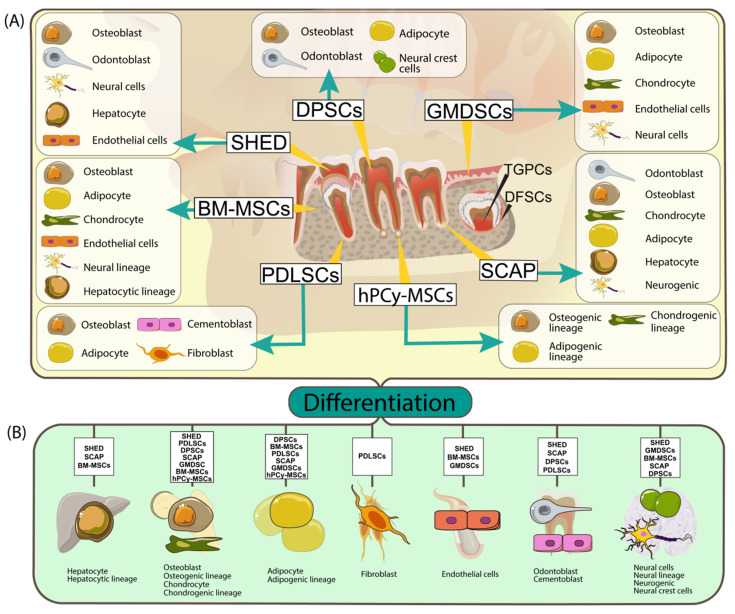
(A) Summary of dental stem cells types, sources and their multilineage differentiation capability (BM-MSCs: bone marrow mesenchymal stem cells; DPSCs: human dental pulp stem cells; SHED: human exfoliated deciduous teeth; PDLSCs: periodontal ligament stem cells; SCAP: stem cells from apical papilla; GMDSC: gingival-mesenchymal derived stem cells, hPCy- MSCs: human periapical cyst-mesenchymal stem cells). The yellow trace lines indicate stem cells origin and the arrows pinpoint their possible differentiation outcome; (B) Through specific environment conditions and different growth factors the dental stem cell types can be differentiated into other cell types (adipocytes, epithelial cells, hepatocyte, and so on).
